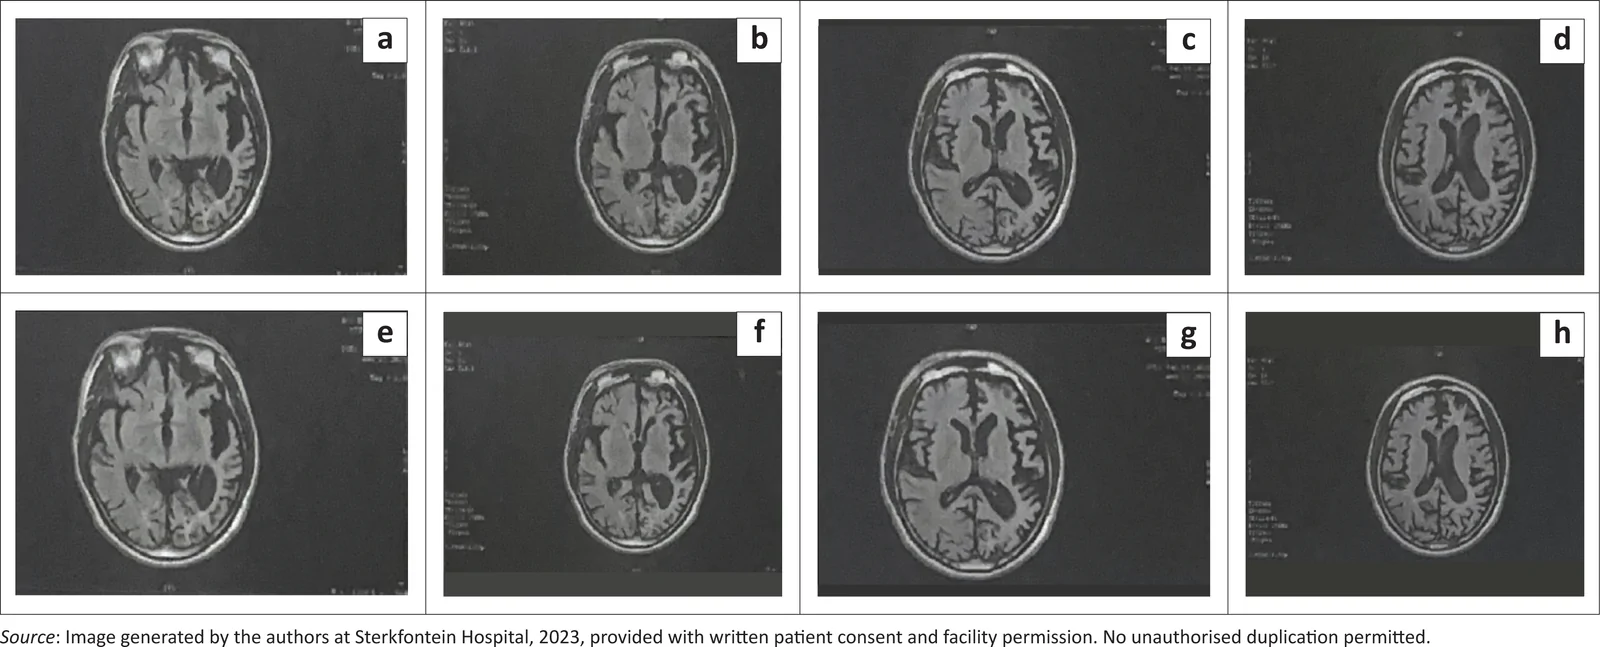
Mr X’s T2 FLAIR axial MRI images (a–h). Significant generalised atrophy, with asymmetric involvement of the left cerebral hemisphere (a–h).
